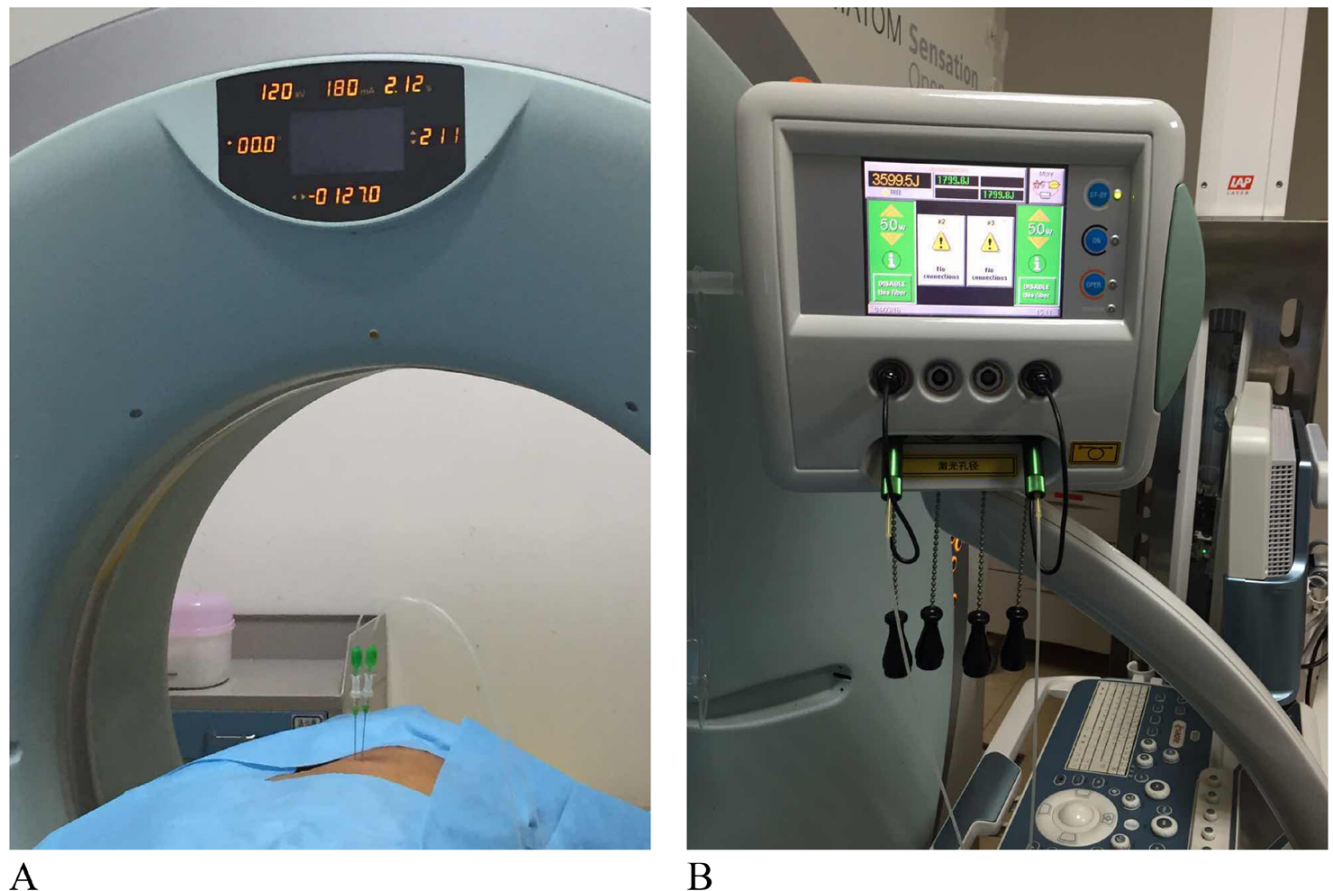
Mechanisms of CT-guided percutaneous laser ablation
